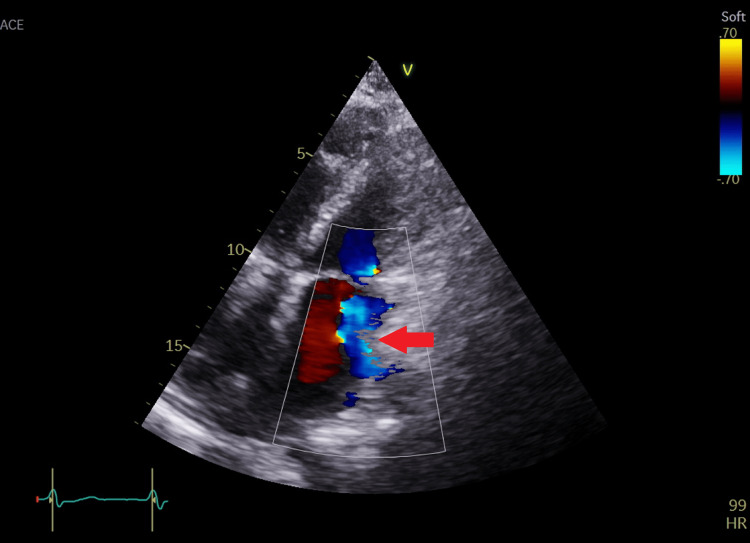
Apical four-chamber view with color coding of the mitral valve showing mild mitral regurgitation.
